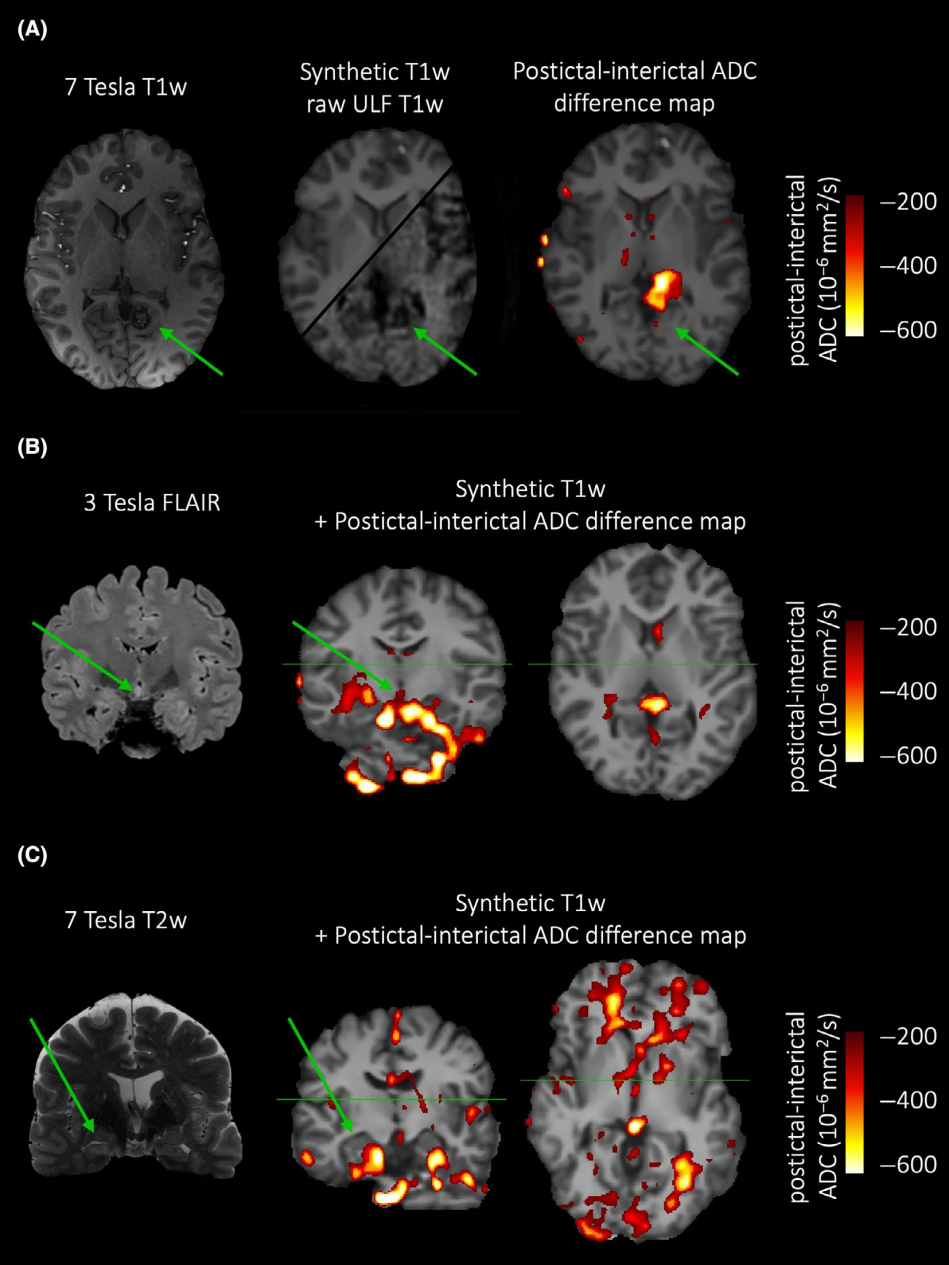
(A) Case 1: 31‐year‐old female with left occipital cavernoma with focal aware seizures; seizure‐scan latency: 29 s. (B) Case 2: 18‐year‐old female with tuber cinereum hamartoma and focal aware gelastic seizures; seizure‐scan latency: 19 min. Please note the postictal diffusion restriction in the splenium of the corpus callosum (right image). (C) Case 3: 53‐year‐old male with right hippocampal sclerosis after a focal to bilateral tonic–clonic seizure; seizure‐scan latency: 5 h 43 min. See Figure S2 for postictal–interictal difference maps with higher and lower ΔADC‐thresholds. ADC, apparent diffusion coefficient; FLAIR, fluid‐attenuated inversion recovery; T1w, T1‐weighted; T2w, T2‐weighted; ULF, ultra‐low‐field.
